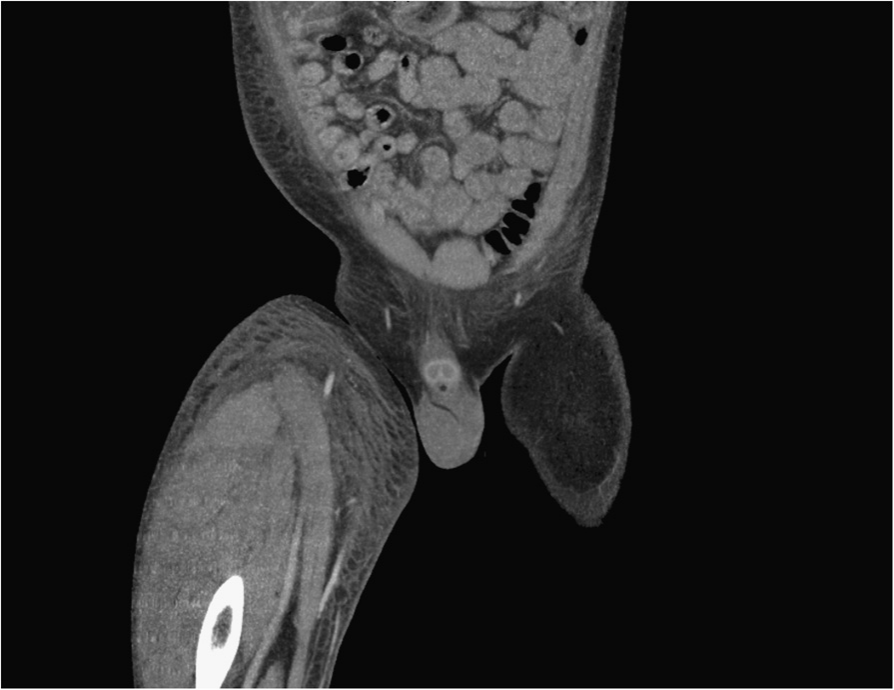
Coronal computed tomography angiogram showing a large hematoma in the lateral component of the thigh.
